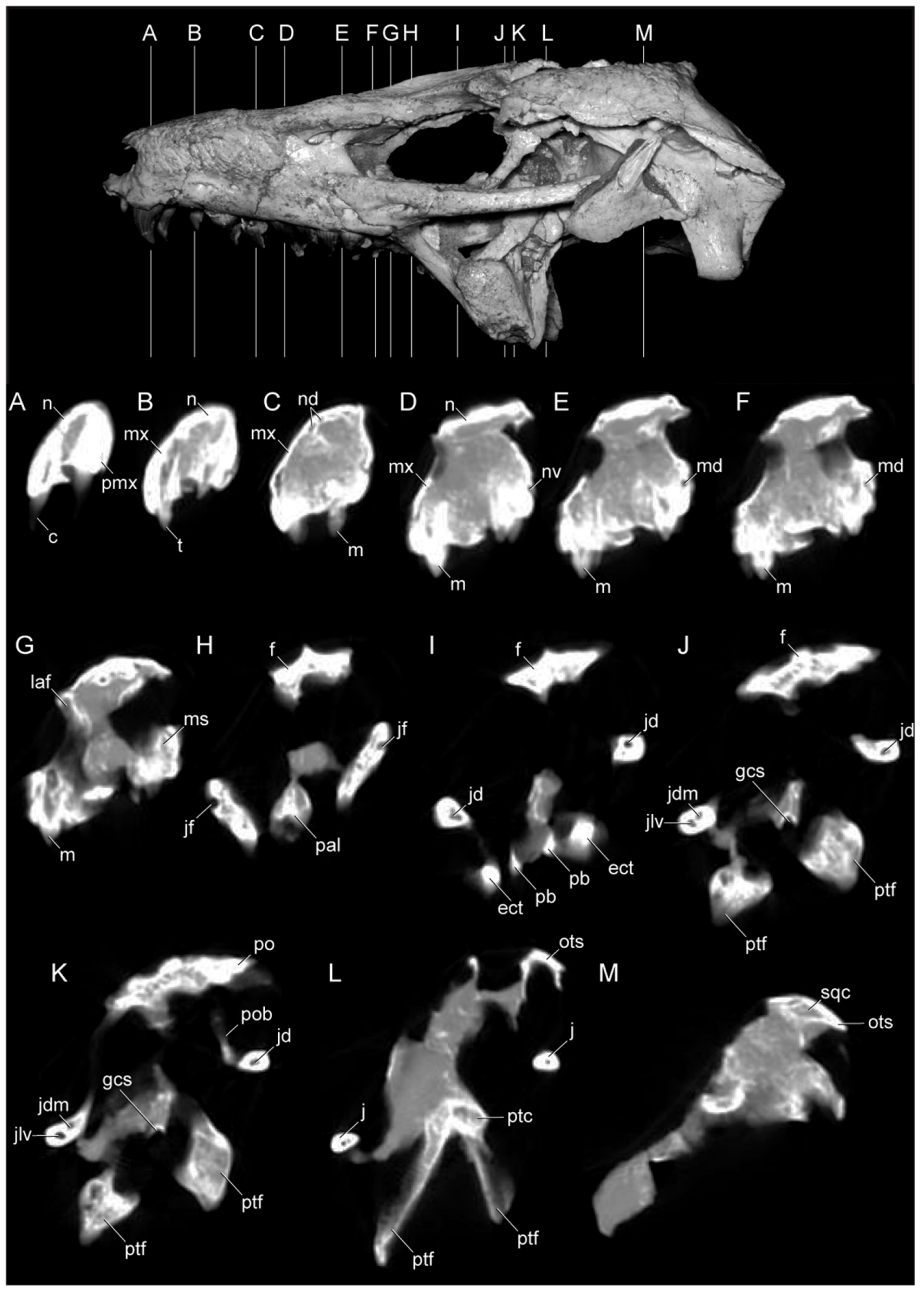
Transversal CT slices of the skull of *Caipirasuchus stenognathus* (MZSP-PV 139) with reference image of the skull showing the placement of the slices A–M. Abbreviations: c, caniniform tooth; ect, ectopterygoid; f, frontal; gcs, grooved choanal septum; j, jugal; jd, jugal neurovascular duct; jdm, jugal dorsomedial duct; jf, jugal foramen; jlv, jugal lateroventral duct; laf, lacrimal foramen; m, molariform tooth; md, maxillary neurovascular duct; ms, maxillary neurovascular sinus; mx, maxilla; n, nasal; nd, nasal neurovascular duct; nv, neurovascular foramen; ots, otic shelf; pal, palatine; pb, palatine bar; pmx, premaxilla; po, postorbital; pob, postorbital bar; ptc, pterygoid internal cavity; ptf, pterygoid flange; sqc, squamosal internal cavity; t, transitional tooth. Scale bar equals 2.5 cm.
